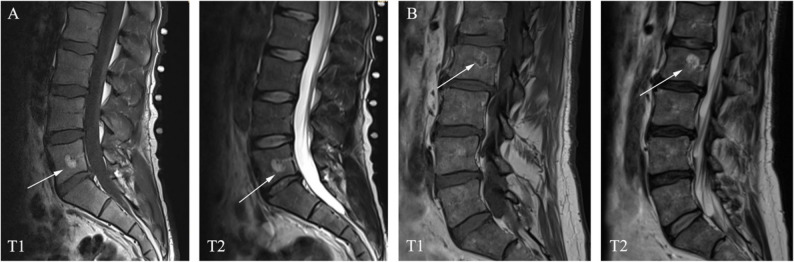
Two distinct types of VHs occurred in the sagittal plane of the lumbar spine. Typical VHs: hyperintense lesion on T1- and T2-weighted images (Figure A); Atypical VHs: hypointense on T1-weighted and hyperintense on T2-weighted (Figure B)
